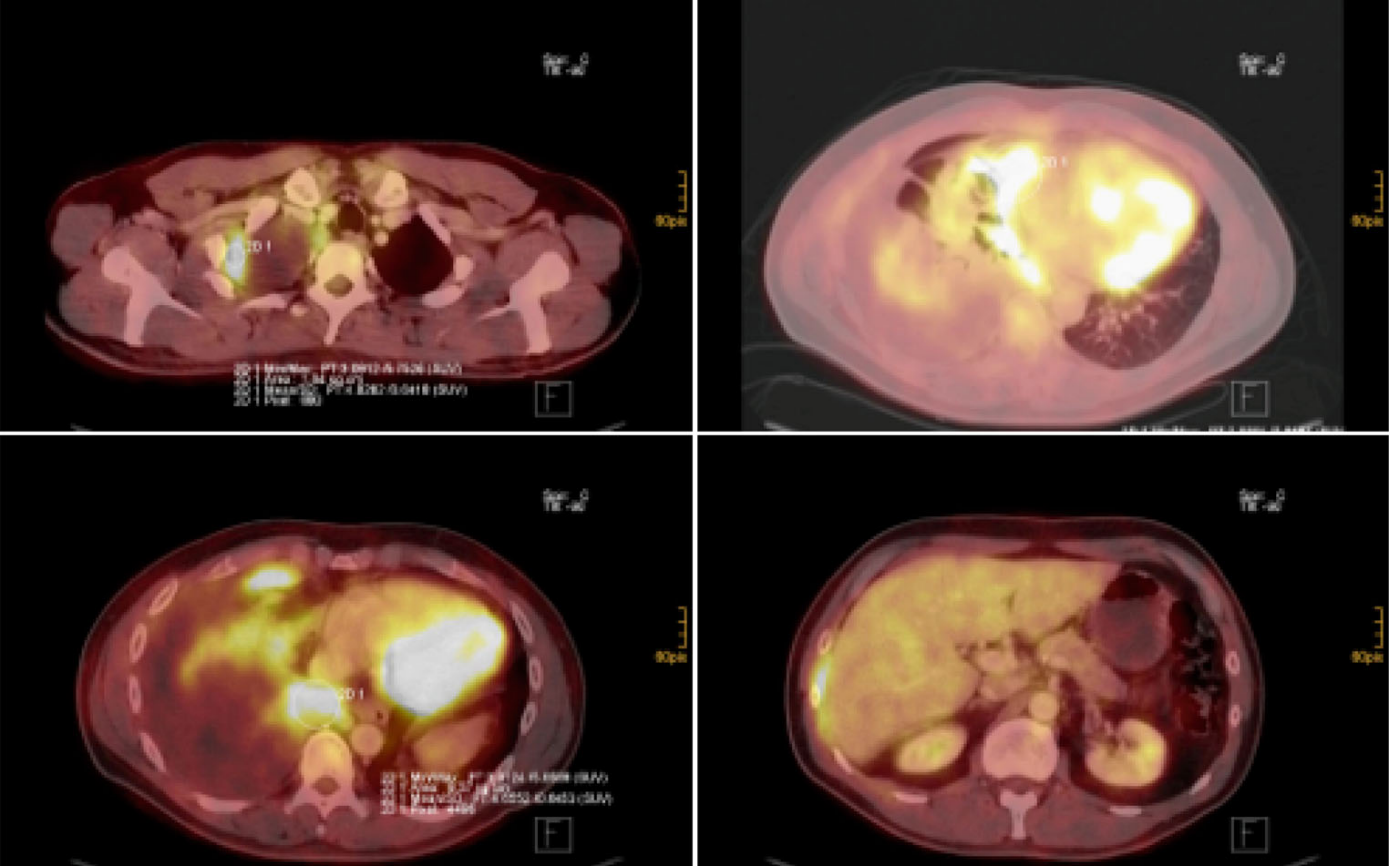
FDG PET-CT images: malignant pleural mesothelioma of the right pleural cavity (various slides of PET/CT fusion imaging). Varoius slides of CT/PET fusion imaging showing pleural tumor apical right (*top left*), involving the visceral and parietal pleura in the pleura costodiaphragmatic area (*bottom left* and *right*) and the pericardium (*top right*)
